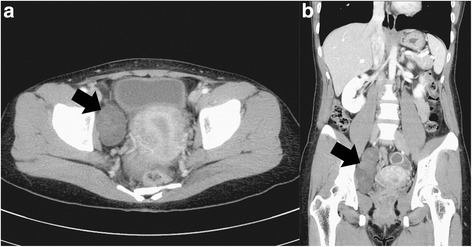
Computed tomography (a) axial and (b) sagittal view showing a right-side heterogeneous pelvic mass posterior to the urinary bladder pushing the uterus to the left side
